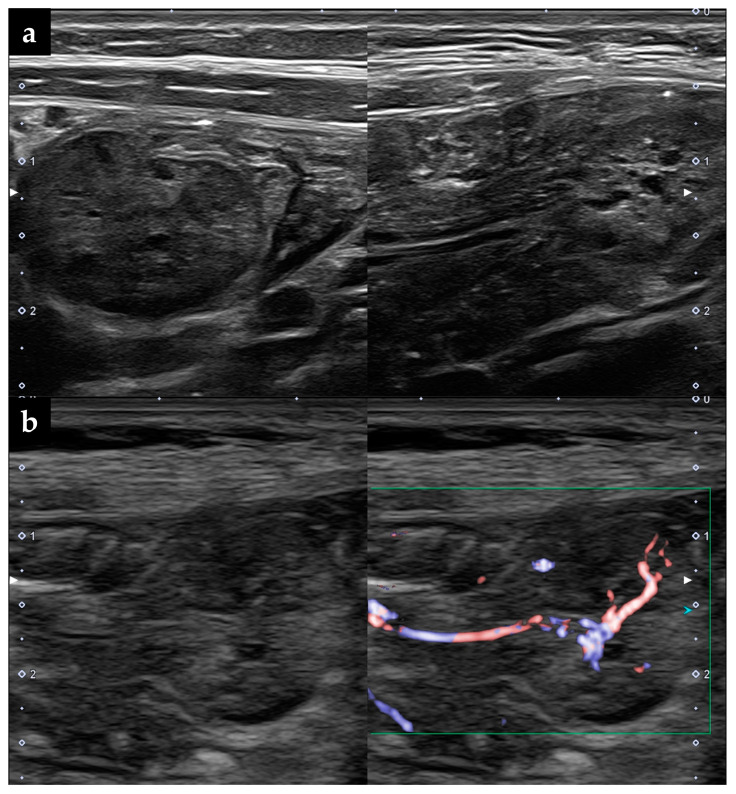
The ultrasonographic findings of the isolated small intestinal polyp. (a) Multiple anechoic cysts are identified within the parenchyma of the polyp. (b) The pedunculated polyp has a diameter of 15 mm and shows increased internal vascularity.
